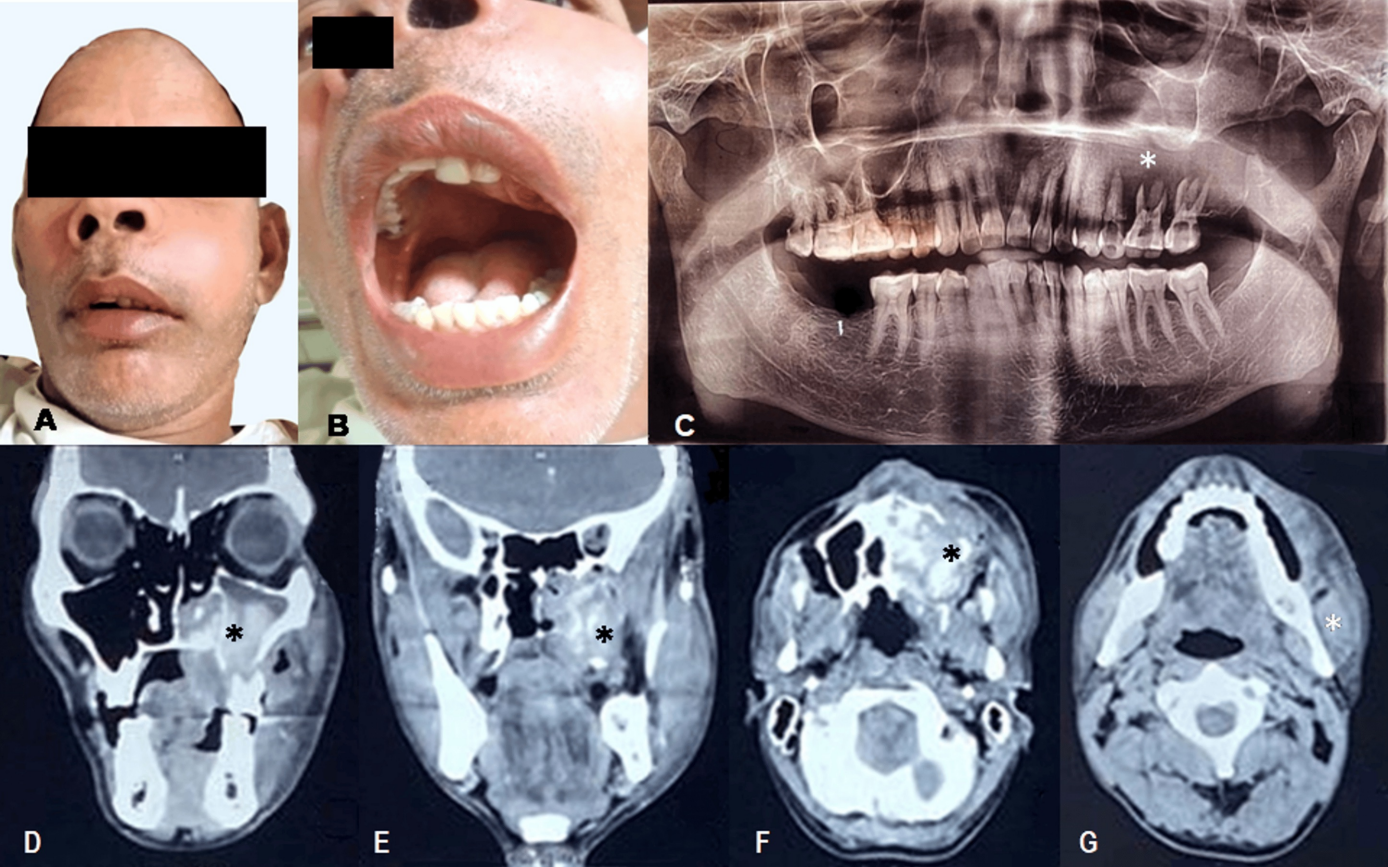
(A) Extraoral view. (B) Intraoral view. (C) OPG showing destruction of the floor of the left maxillary sinus (asterisk). D-G: CBCT of the head and neck findings. (D) CT image demonstrating an invasive, heterogeneously enhancing mass in the left parotid gland spreading into the sinus with the expansion of the left maxilla. (E) Ill-defined heterogeneous mass occupying the left maxillary sinus. (F) Poorly defined radiolucency with maxillary cortical expansion. (G) Tumor in relation to the left ramus and mandible (asterisk). OPG: orthopantomogram; CBCT: cone-beam computed tomography
